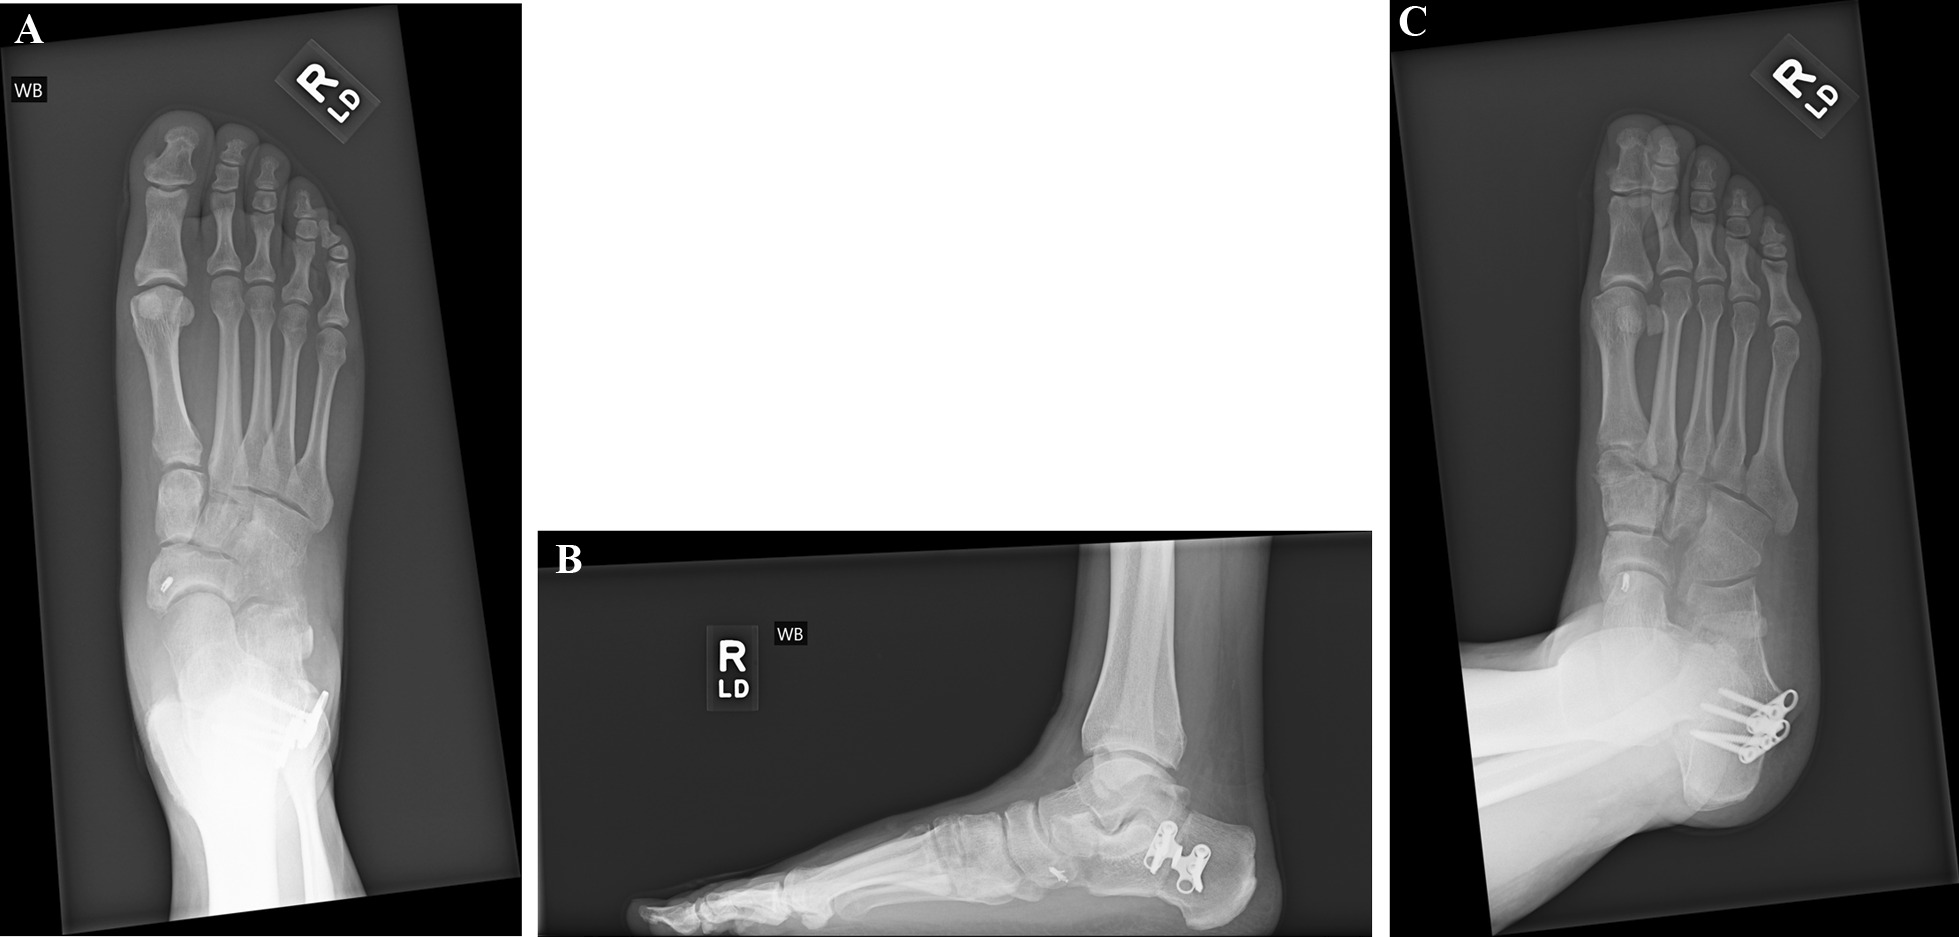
A Postoperative plain anteroposterior radiograph; patient is status post-resection of cuboid-navicular coalition, MDP, Evans osteotomy, Cotton osteotomy, Kidner procedure, and an endoscopic gastrocnemius recession. B Postoperative plain medial oblique view. C Postoperative plain lateral view
